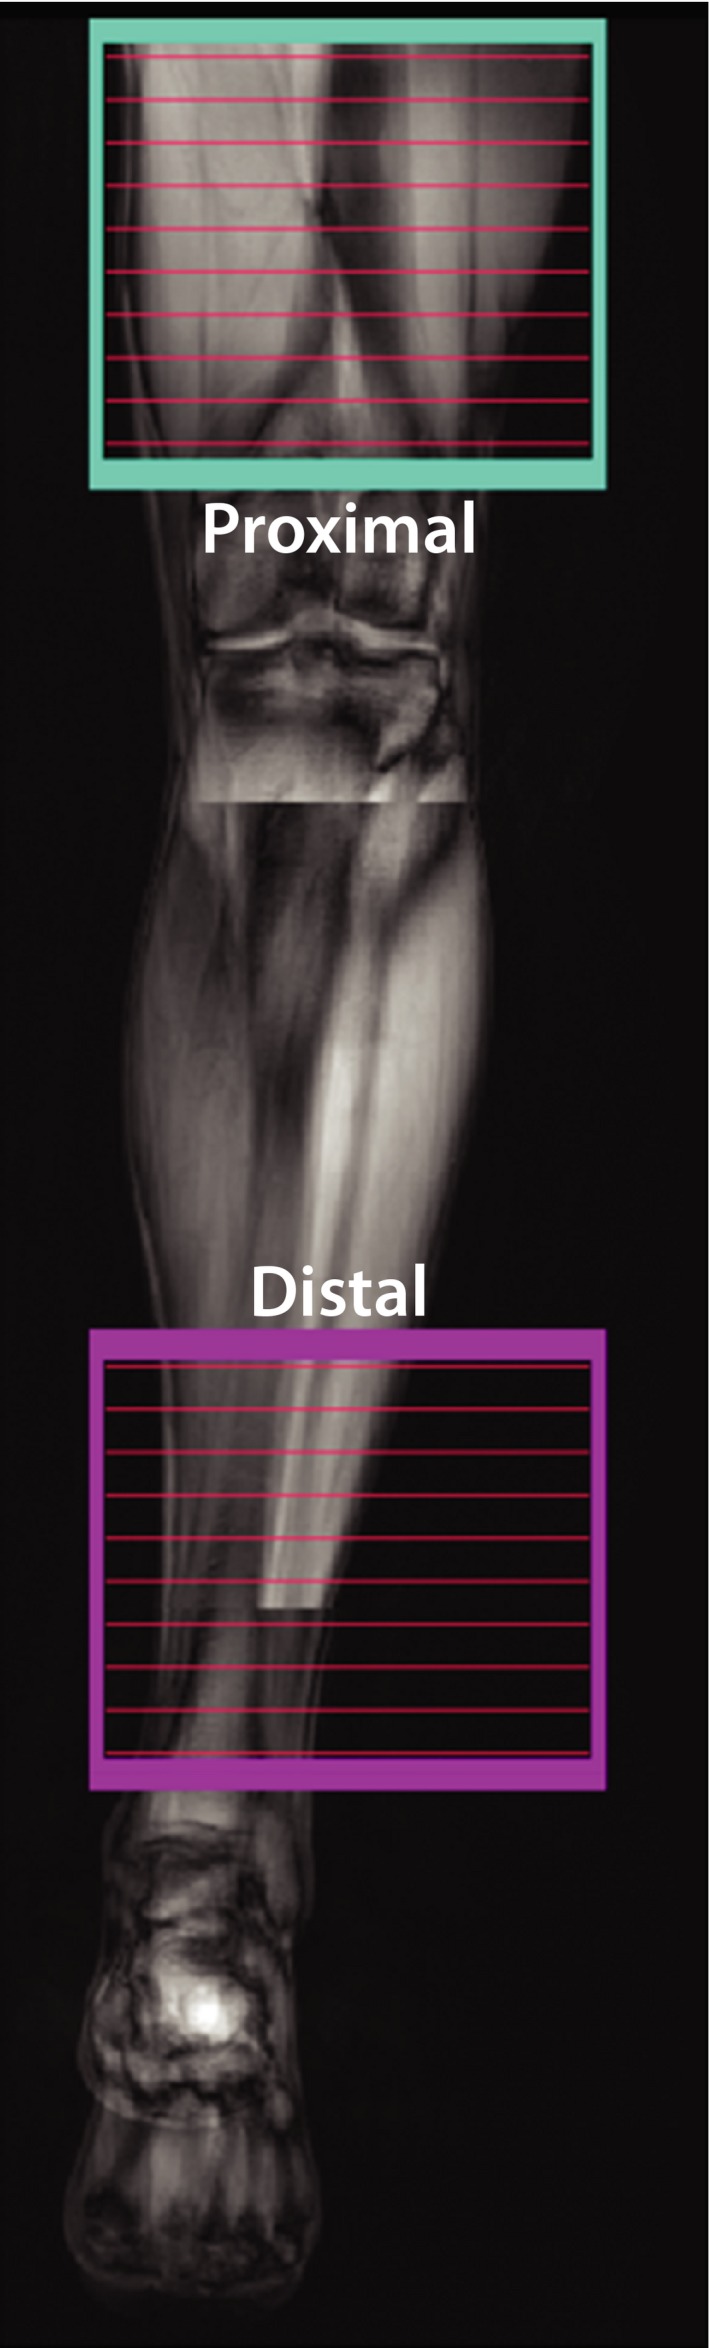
MRI volumes used for leg scan. The proximal leg area is the top, seafoam‐colored box and the distal leg area is the bottom, magenta‐colored box. Red lines inside indicate slice selection.
